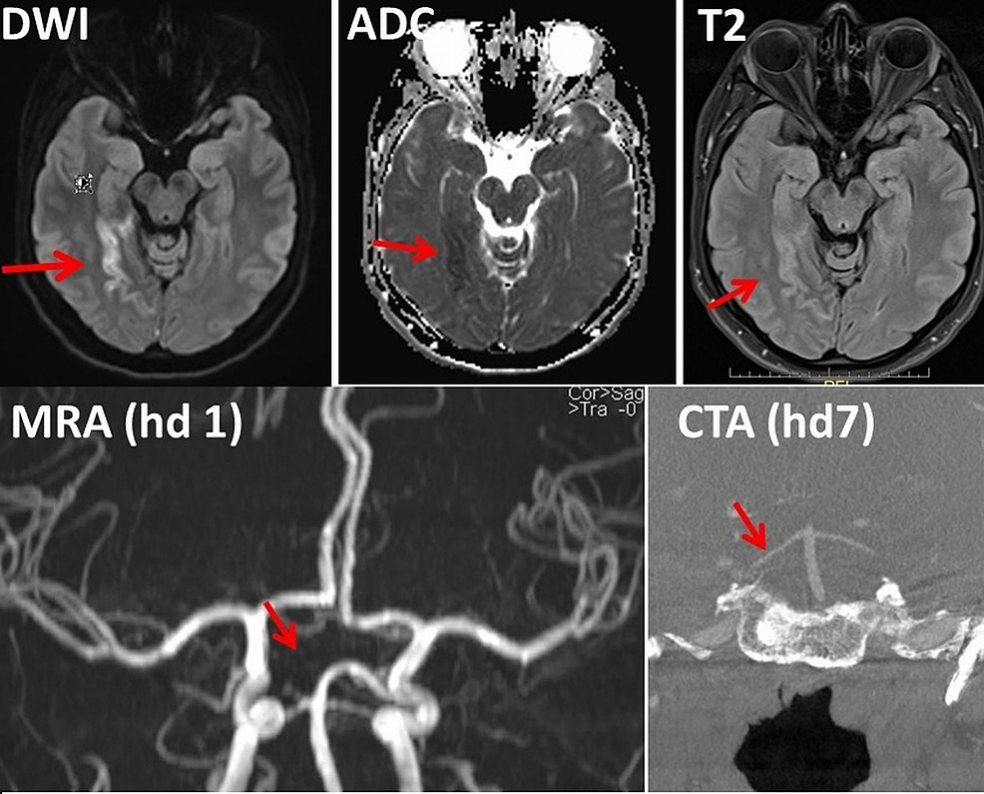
Cerebral MRI and CT angiography of the index patient. MRI showing an ischemic stroke in the territory of the right posterior cerebral artery (upper panels). Magnetic resonance angiography (MRA) on admission shows discontinuation of the right P1 segment (lower left panel). Normal flow in both posterior cerebral arteries (PCAs) was documented on CT angiography (CTA) after seven days of nimodipine, suggesting vasospasm. DWI: Diffusion-weighted imaging, ADC: Apparent diffusion coefficient.
